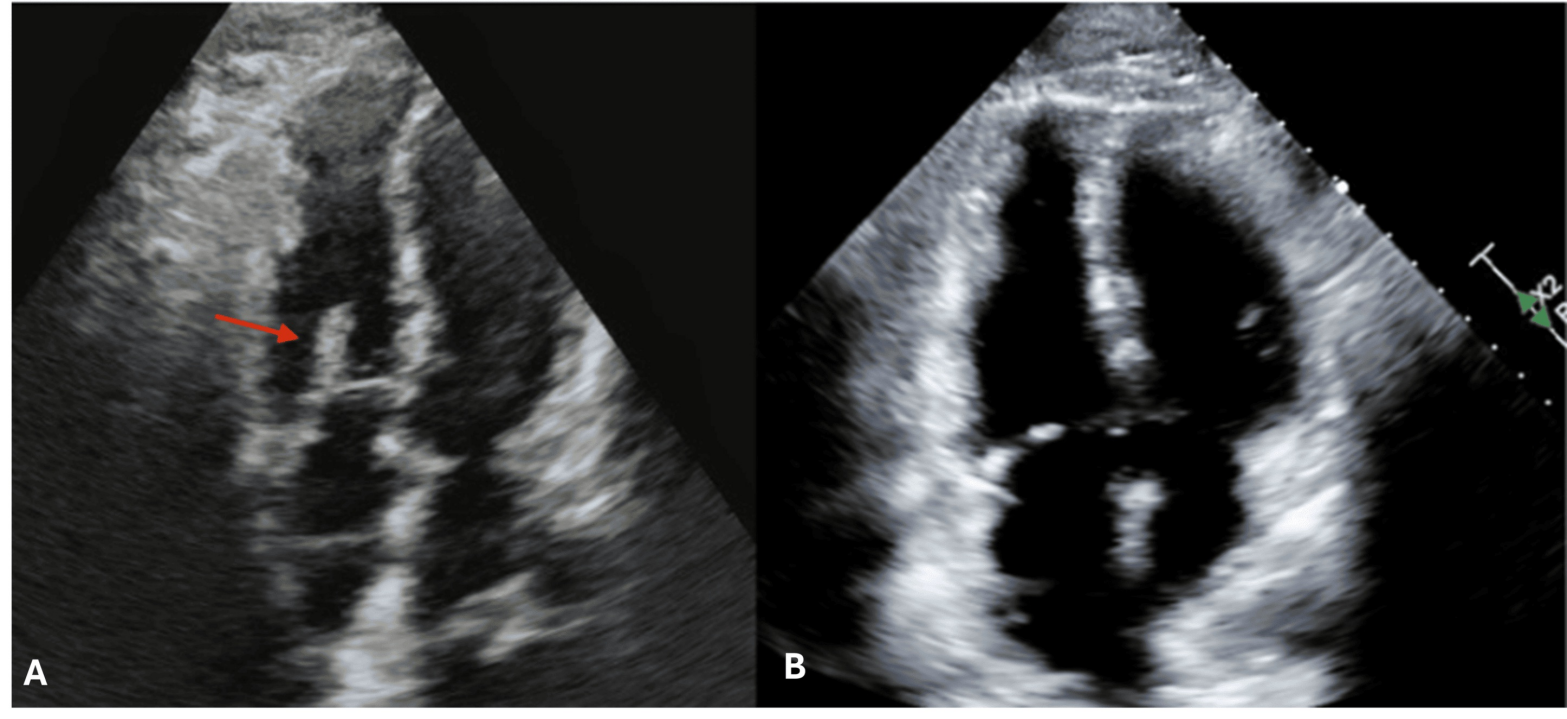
Transthoracic echocardiograms (A) Transthoracic echocardiogram with intracardiac thrombus (red arrow) prior to lytic infusion; (B) Transthoracic echocardiogram with no apparent thrombus after lytic infusion.
